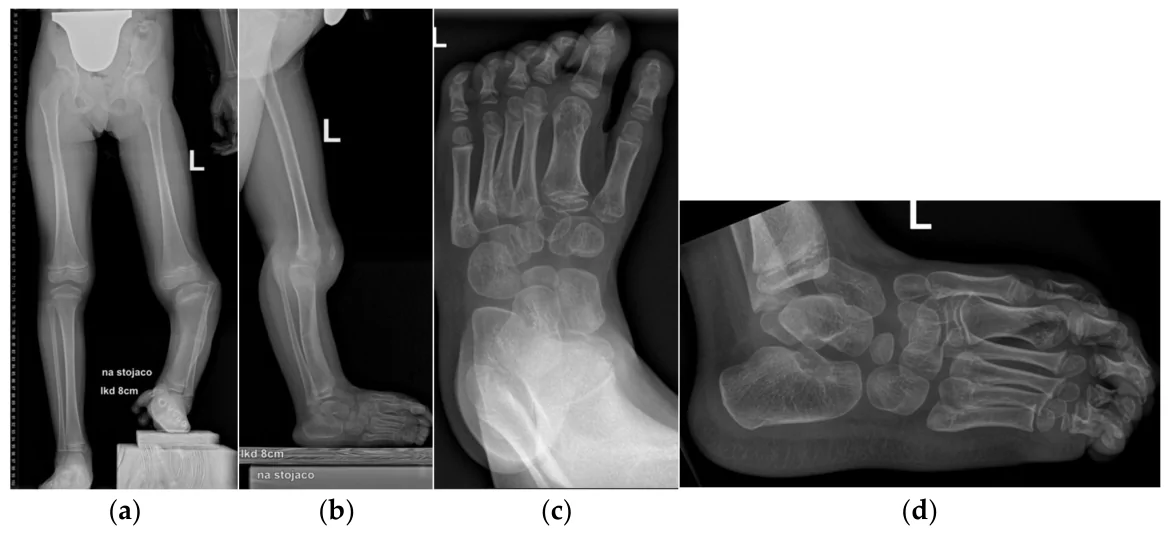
Radiographic views of type IIB TH: (a) knee varus with subluxation and 8 cm shortening; (b) fibular overlengthening; (c) varus foot deformity; (d) accessory toe ray with double talus. lkd—left lower limb, na stojąco—standing position, L—left.
